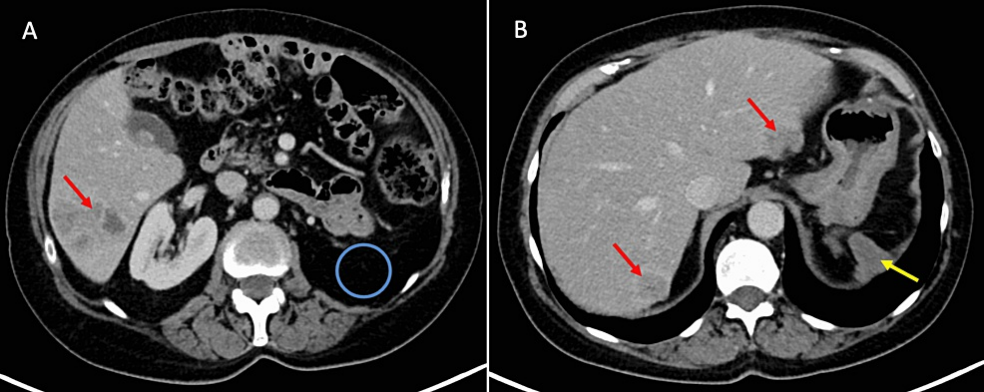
Portal-phase CT of the abdomen prior to SIRT therapy. (A) Segment 6 liver metastasis (red arrow) with an absence of the left kidney in the left renal bed (blue circle) after left adrenalectomy and nephrectomy. (B) Segment 2 and segment 7 liver metastases (red arrows). A haematoma of the resection bed (yellow arrow) was present. This was deemed stable with subsequent imaging. CT: computed tomography; SIRT: selective internal radiation therapy
